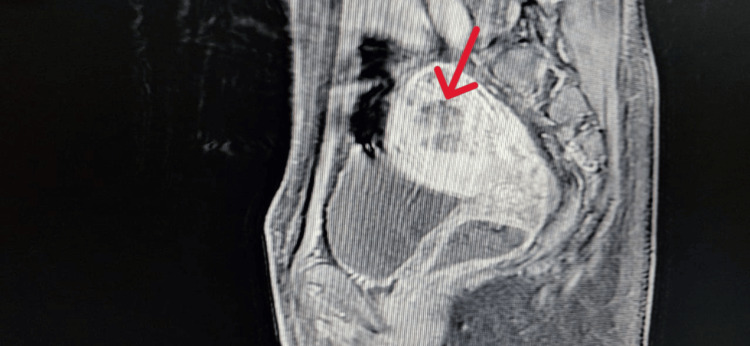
MRI sagittal T1-weighted image showing a globular uterus with a posterior and left lateral corporeal multilocular mass that is poorly defined and hypointense (red arrow).
